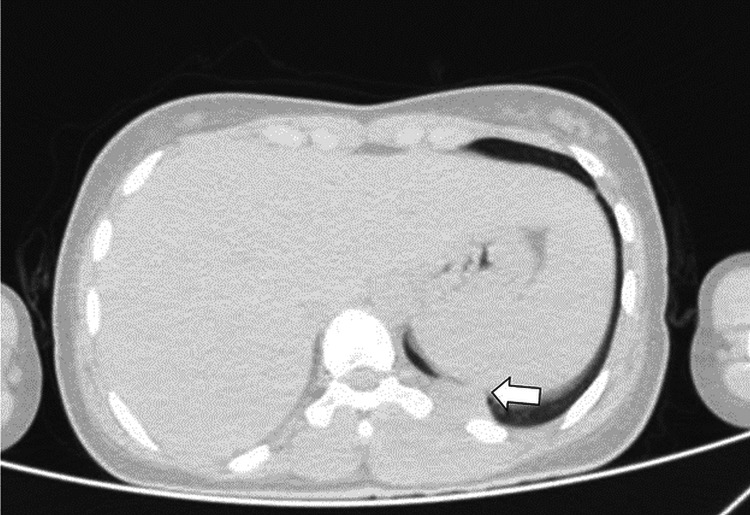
CT scan of the mass Axial non-contrast CT shows a mass (arrow) arising from paravertebral muscles and thoracic soft tissue with involvement of the left pleura. Note that there is no rib erosion.
